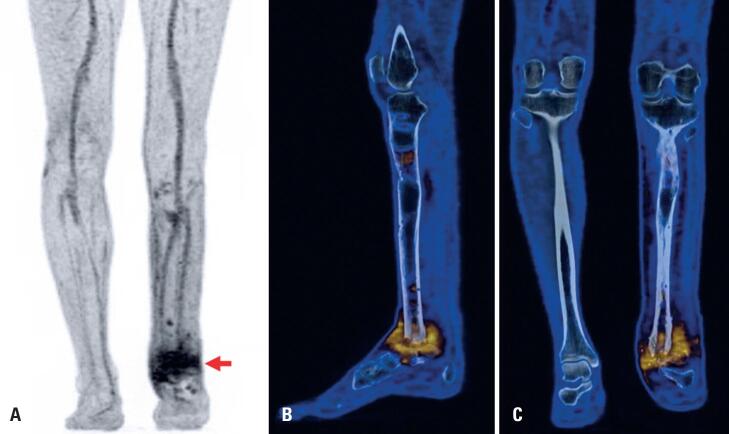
[^68^Ga]Ga-DOTA- Ubiquicidin_[29-41]_ PET/CT of a 39-year-old man with chronic osteomyelitis in the left distal tibia and ankle joint. Whole-body PET/CT imaging was performed 1 h after intravenous tracer injection (250 MBq) on a Biograph mCT scanner (Siemens Medical Solutions, Germany). PET data were acquired for 4 min per bed position. (A) The maximum intensity projection (anterior view), (B) Fused PET/CT sagittal slice of the left leg and (C) Fused PET/CT coronal slice of the legs show tracer abnormal uptake in the distal portion of the left tibia and talus and in periarticular soft tissue
